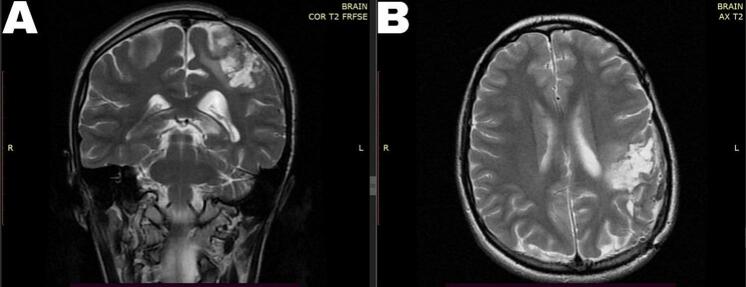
Post-operative brain MRI after six months. (A) T2 Coronal, (B) T2 Axial. Showing good redistribution of the brain without any signs of recurrence.
